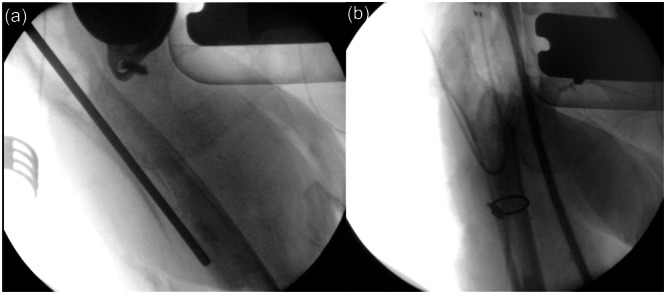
(a) The intraoperative X‐ray shows a perforation of the femoral shaft during ultrasound‐guided cement removal. (b) After complete removal the bone cement, the fracture was stabilised using a cerclage wire in a Girdlestone situation.
